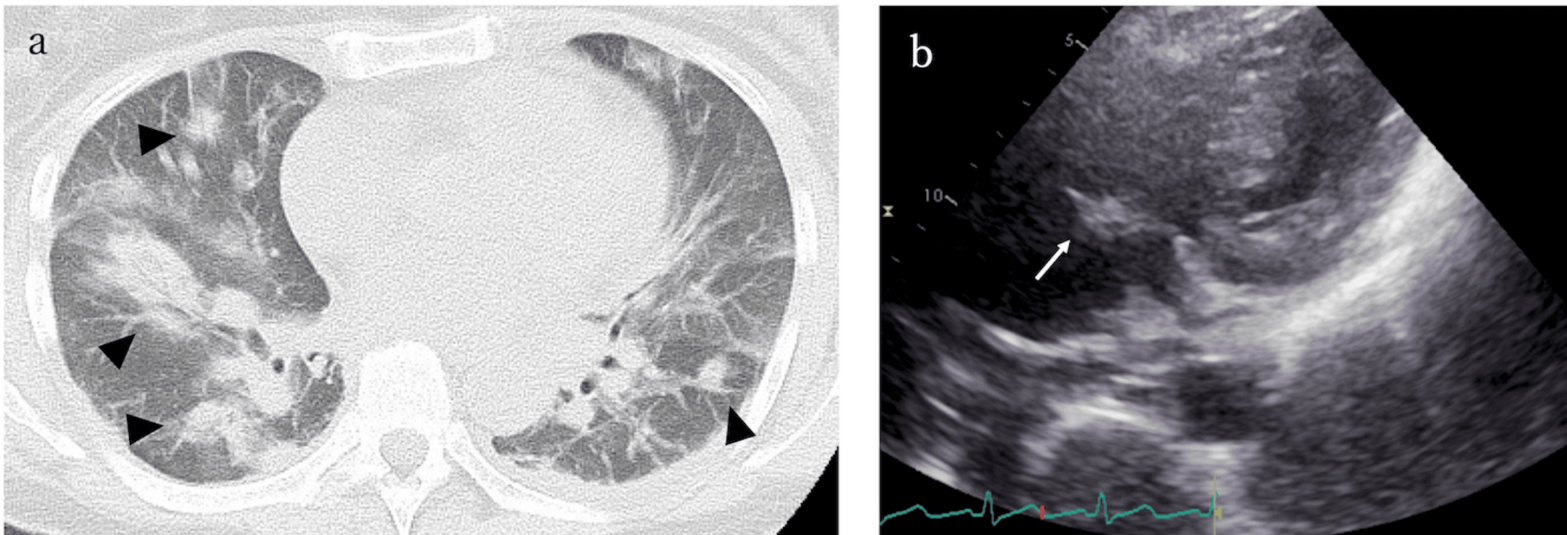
Imaging findings (a) Plain chest computed tomography showing multiple nodular shadows in the lungs. (b) Echocardiogram showing a vegetation attached to the tricuspid valve
